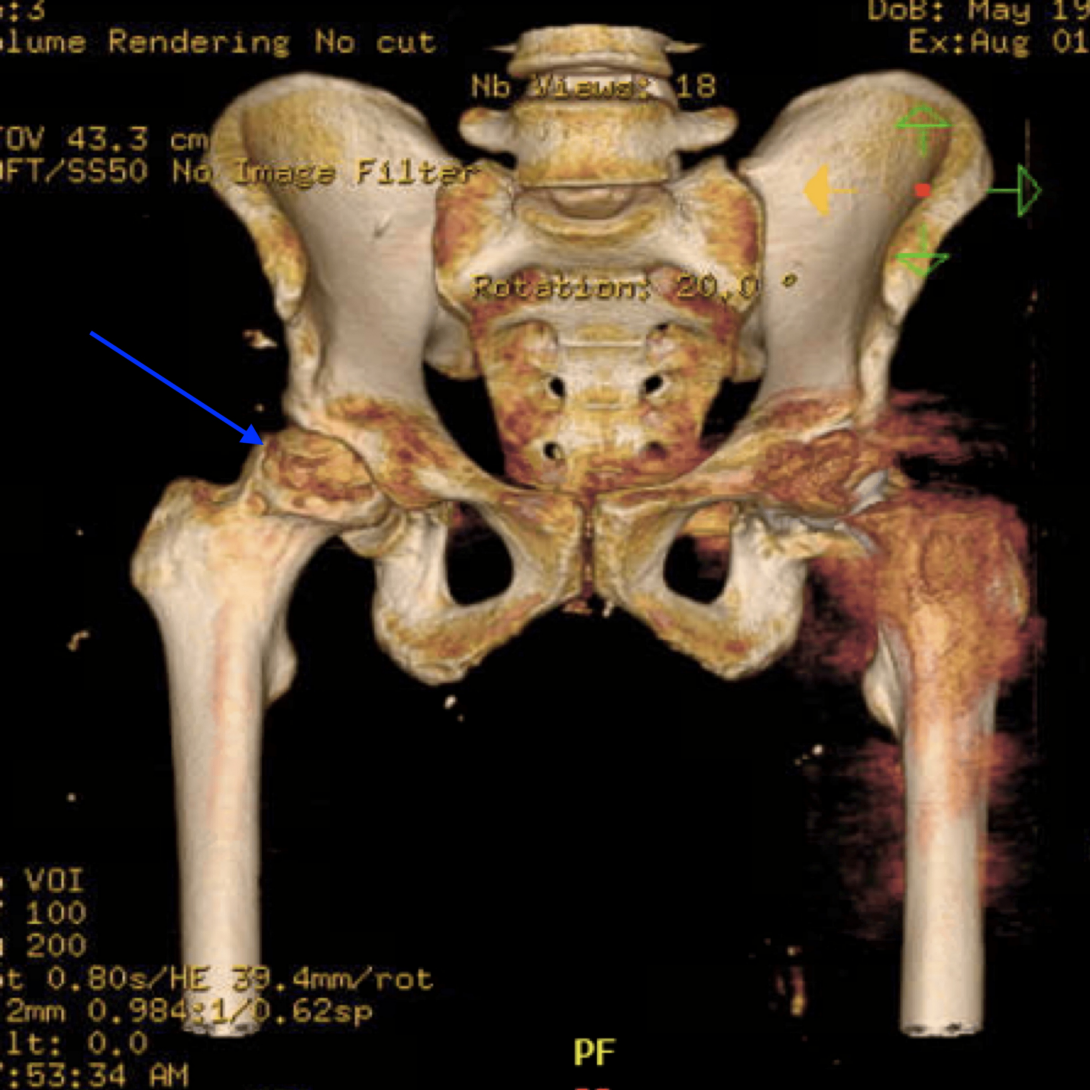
CT scan showing osteolytic lesions in the right femoral head
